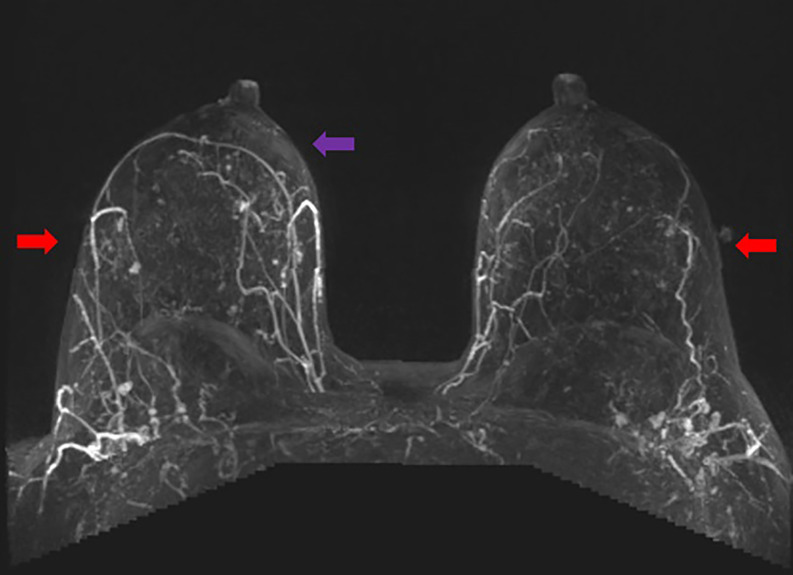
Axial MRI maximum intensity projection (MIP) images. The lateral mammary vessels are more dominant (red arrows) and extend further to the nipple areola complex than the medial perforators off the internal mammary vessels (purple arrows) which do not quite reach the nipple areola complex on either side. Therefore, in this case, the lateral blood supply was selected to keep the nipple areola complex alive.
